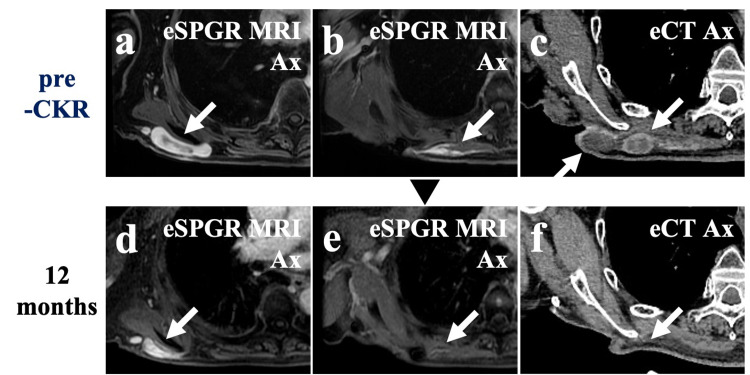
MRI and CT before and after CKR (a), (b), and (c) are pre-CKR images. (d), (e), and (f) are images 12 months after CKR. (a), (b), (d), and (e) are enhanced SPGR MRIs. (c) and (f) are enhanced CT (a). Enhanced lesions (white arrow)
